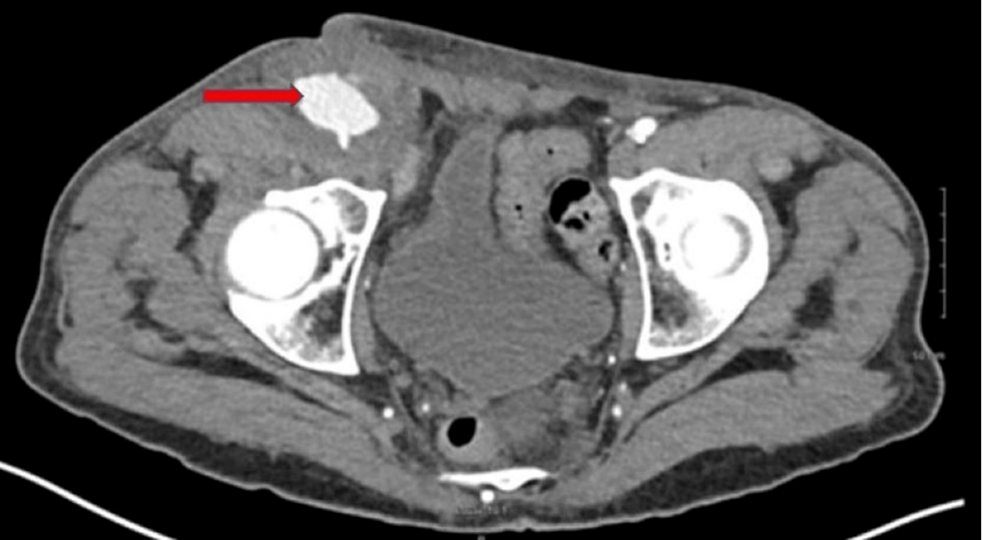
CT angiography demonstrating pseudoaneurysm formation.
